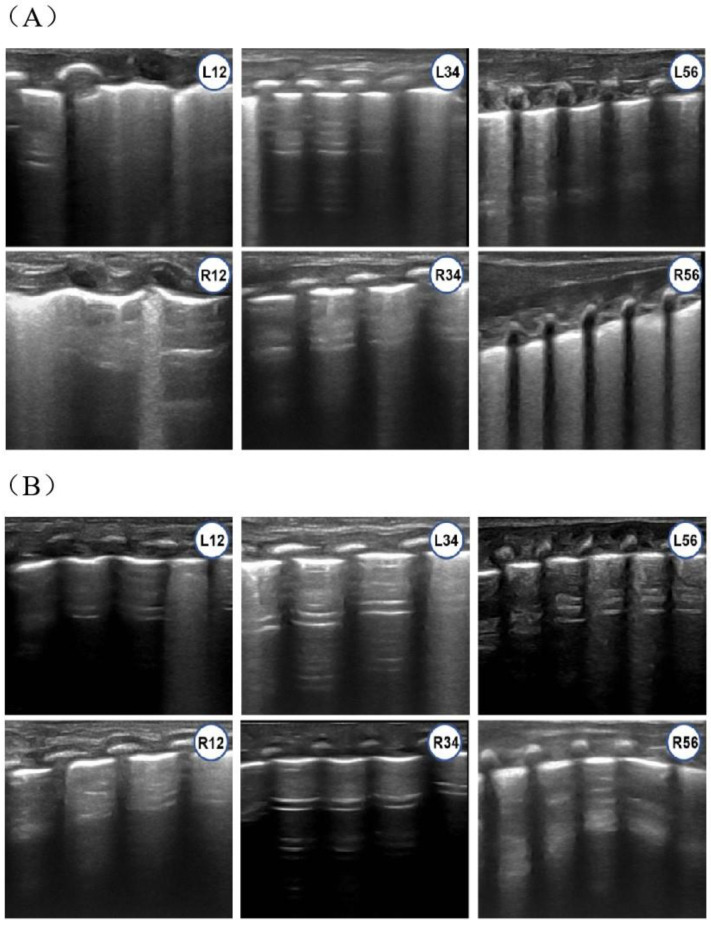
Lung Ultrasound Findings of an Infant with pulmonary edema (Lung Ultrasound Score = 9). (A) A preterm infant born at a gestational age of 31 + 5 weeks underwent lung ultrasound at 1 h of postnatal age. The findings showed both the disappearance of A-lines and the presence of an AIS (alveolar interstitial syndrome) pattern in regions L12, L56, R12, and R56. B-line occupying more than 50% of the lung fields in these regions, each lung fields LUS was 2. Regions L34 showed A-lines occupied more than 50% of the lung fields and AIS occupied two intercostal spaces, LUS was 1. Regions R34 showed A-lines in each intercostal spaces with sporadic B-lines, LUS was 0. No lung consolidation was observed in any lung field. Although the total LUS was 9, the findings were not consistent with NRDS. The diagnosis was pulmonary edema and PS was not administered. (B) Lung ultrasound re-examination at 24 h of postnatal age of this infant showed clear A-lines in all lung fields, a small number of B-lines, and only a few intercostal spaces with an AIS pattern. No lung consolidation was observed.
